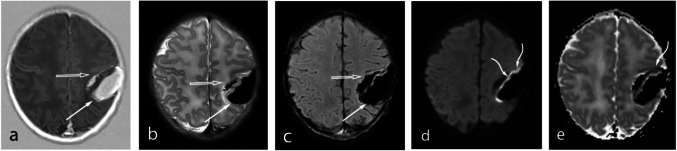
Neonatal subpial hemorrhage with underlying cortical infarct and small white matter hemorrhage. Brain MRI in a 5-day-old term male with seizures (a-e). Axial T1-weighted image (a) shows high signal intensity, T2-weighted (b) and susceptibility-weighted (c) images show low signal intensity of subpial hemorrhage on left parietal lobe overlying cortex and the precentral gyrus (arrows). Axial DWI (d) and ADC (e) maps show decreased diffusivity indicating underlying cortical infarct (curved arrows). Note small hemorrhage in the underlying white matter (open arrows in a, b, c)
